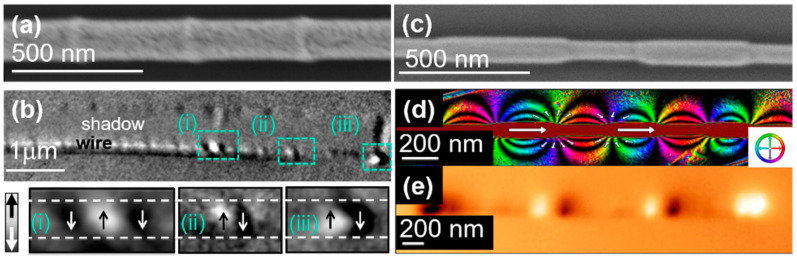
(a) SEM image of a bamboo FeCoCu nanowire, (b) XMCD-PEEM image of bamboo-type FeCoCu nanowire (a) oriented perpendicular to the incident X-rays (Adapted with permission from ref. [21]. Copyright 2016 Royal Society of Chemistry). The insets show a closer view at the local magnetic configuration marked in (b) by green dashed squares, (c) SEM image of a modulated FeCoCu nanowire, (d) magnetic flux images of modulated FeCoCu nanowire (c) reconstructed from the magnetic phase shift images, (e) MFM image of a modulated FeCoCu nanowire. Adapted with permission from ref. [22]. Copyright 2016, American Chemical Society.
